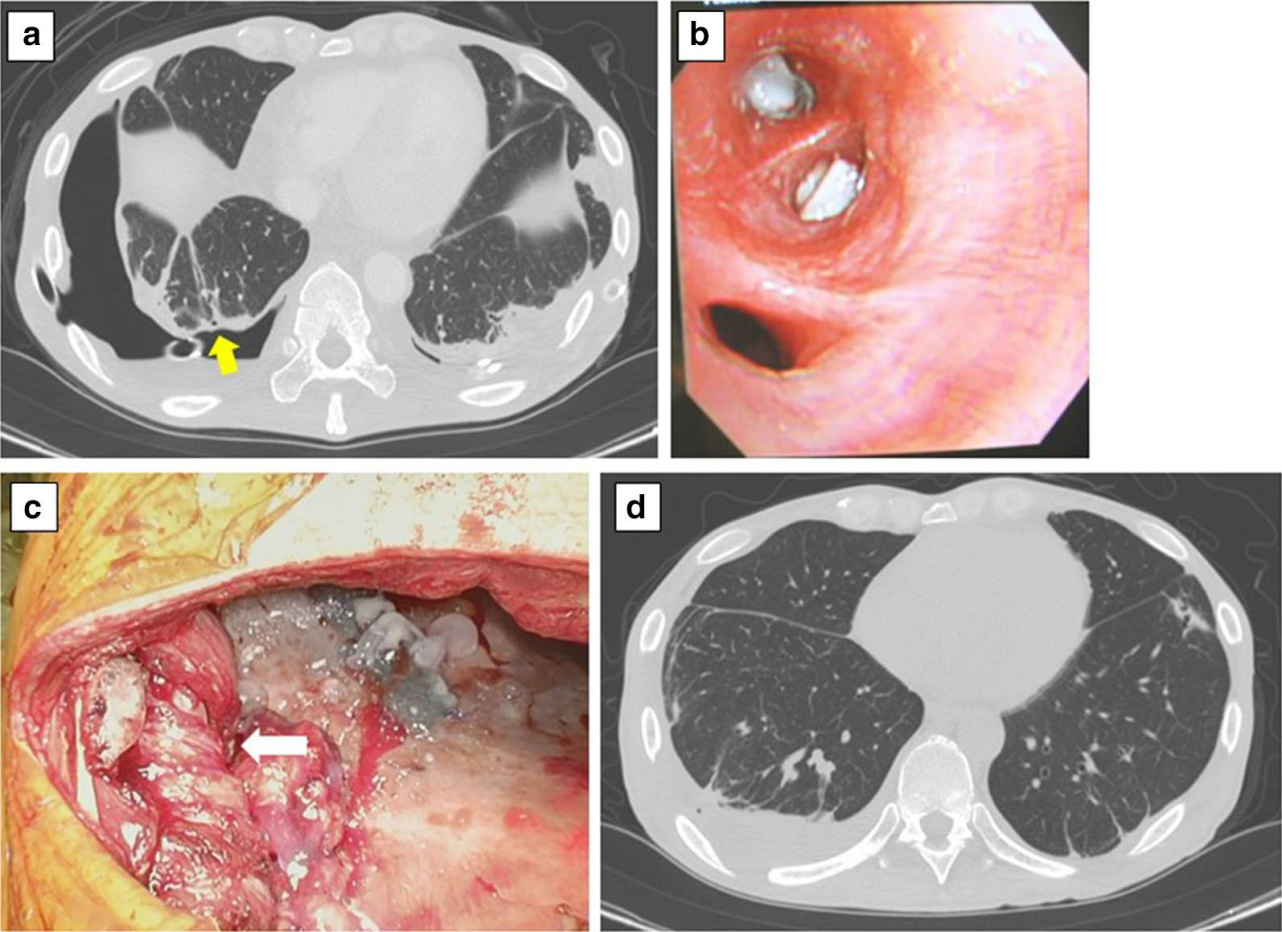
a CT after the first operation revealed a bronchopleural fistula in the right pleural cavity. The right B^10^c was thought to be the responsible bronchus (yellow arrow). b Endobronchial Watanabe spigots were inserted into the right B^10^b and B^10^c bronchi. c The right S^10^ fistula was superimposed and closed using a pedicled intercostal muscle flap (white arrow). d CT after the second operation showed that both sides of the lung expanded without residual pleural space
